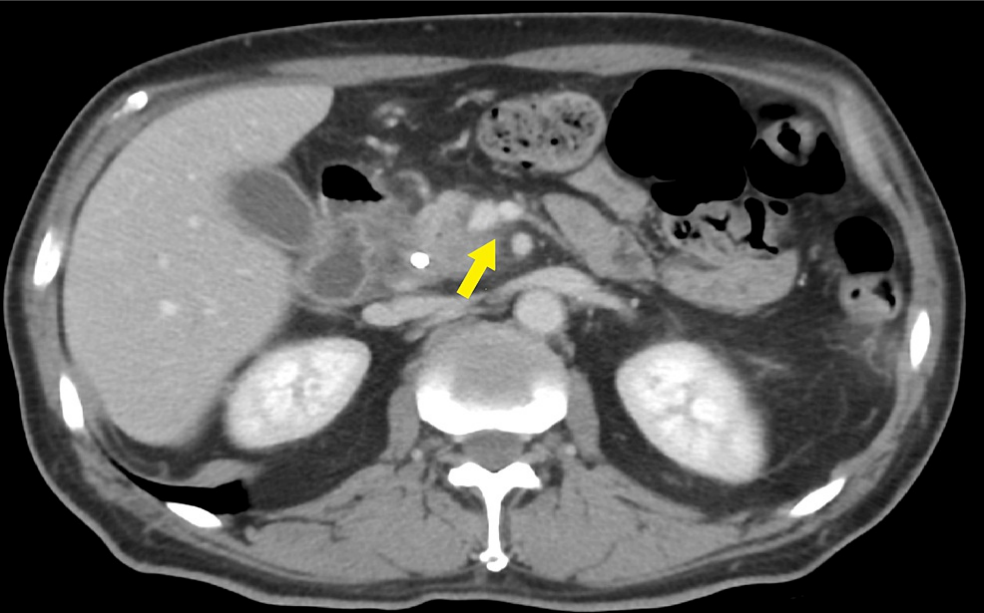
Another axial CECT image showing the infiltrative hypodense pancreatic head lesion CECT: contrast-enhanced computed tomography
